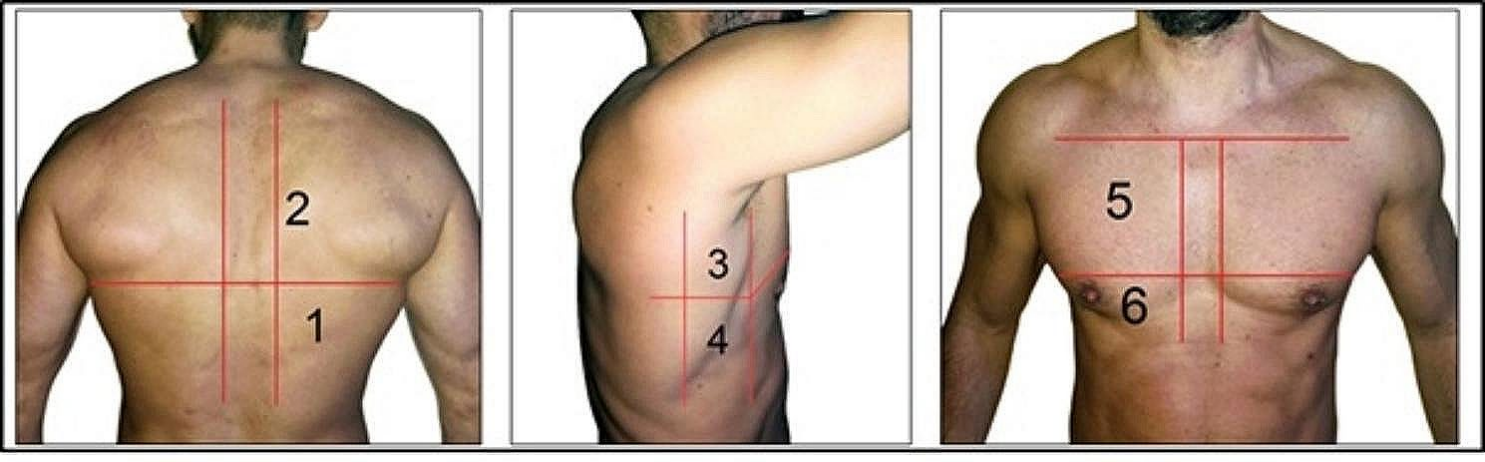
Region of interest for lung ultrasound score
